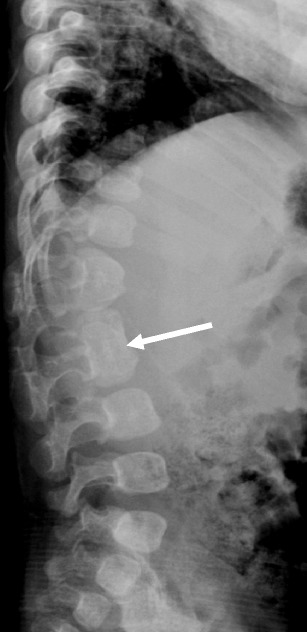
Ventral block vertebra in 8 month old: Lateral radiograph of the spine demonstrates fused L2 and L3 vertebral bodies (*arrow*). The T12 and L1 vertebral bodies were also partially fused
